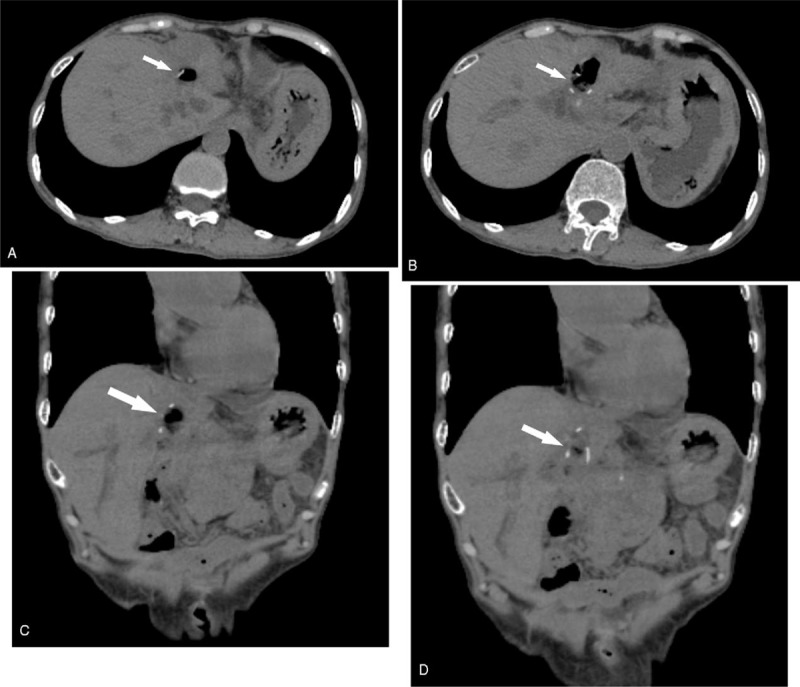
Computed tomography (CT) scan showed high density shades on the duodenal wall (arrow).
